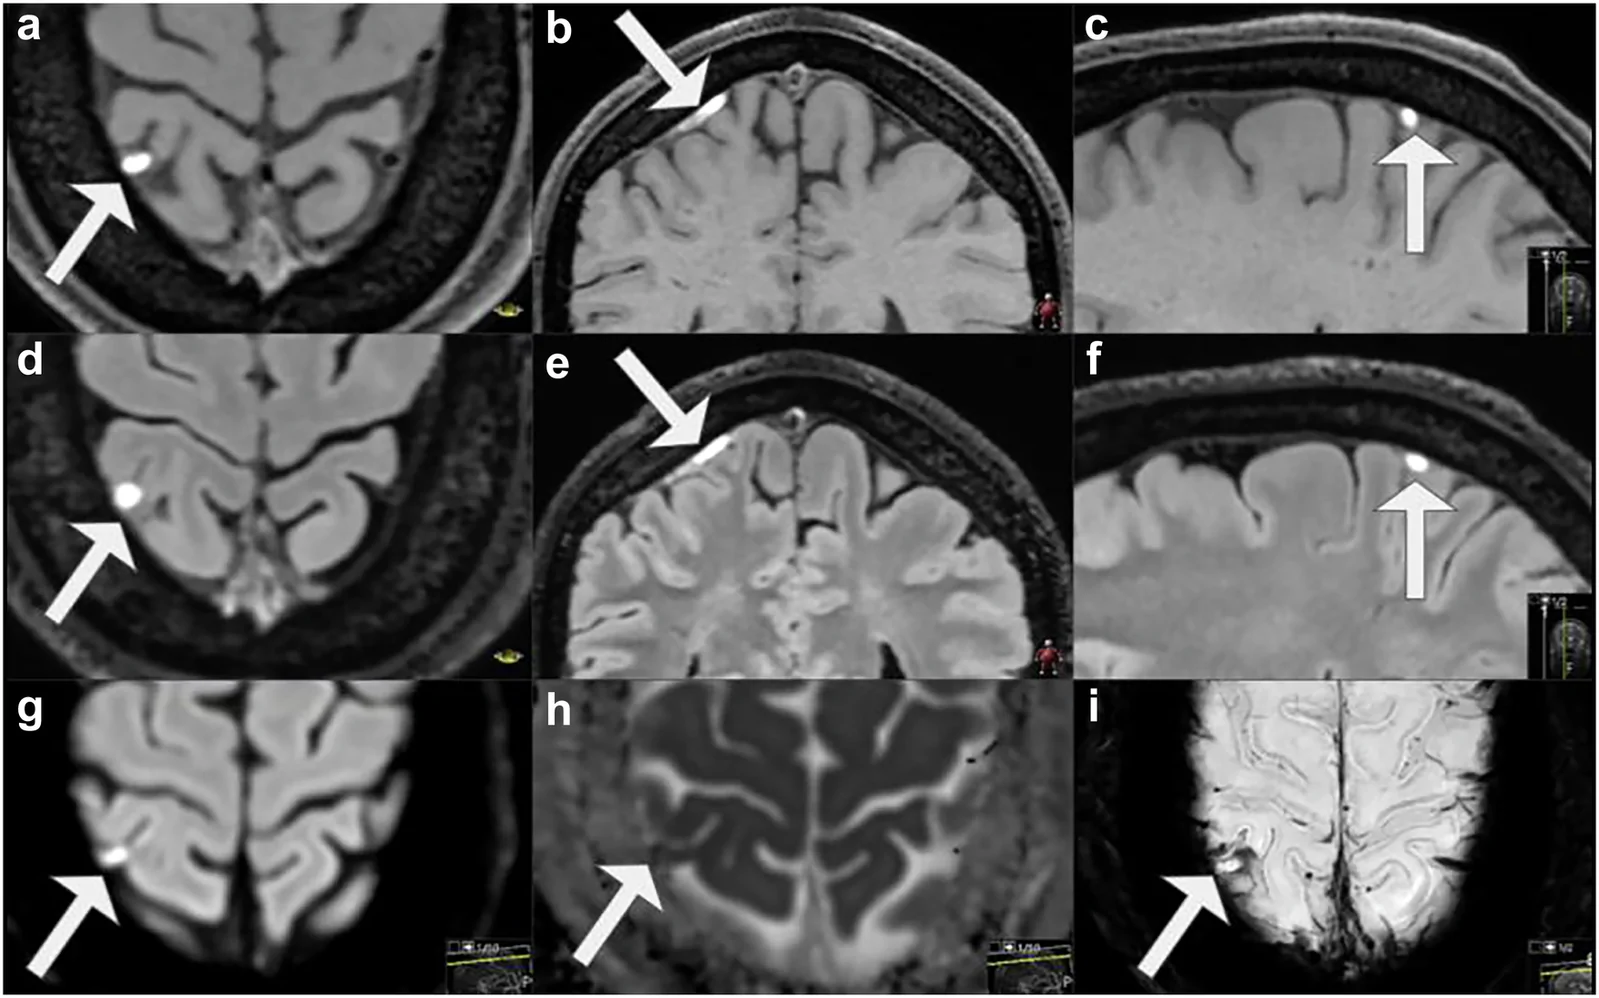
Right parietal cortical vein thrombosis on multisequence MRI. Upper row (a–c): T1-weighted images (axial, coronal, sagittal) show intrinsic hyperintensity in the thrombosed cortical vein (arrows). Middle row (d–f): T2-FLAIR (axial, coronal, sagittal) demonstrates hyperintense thrombus (arrows). Lower row: (g) DWI hyperintensity, (h) ADC hypointensity confirming restricted diffusion, and (i) SWI central hyperintensity (arrows), collectively confirming cortical vein thrombosis
